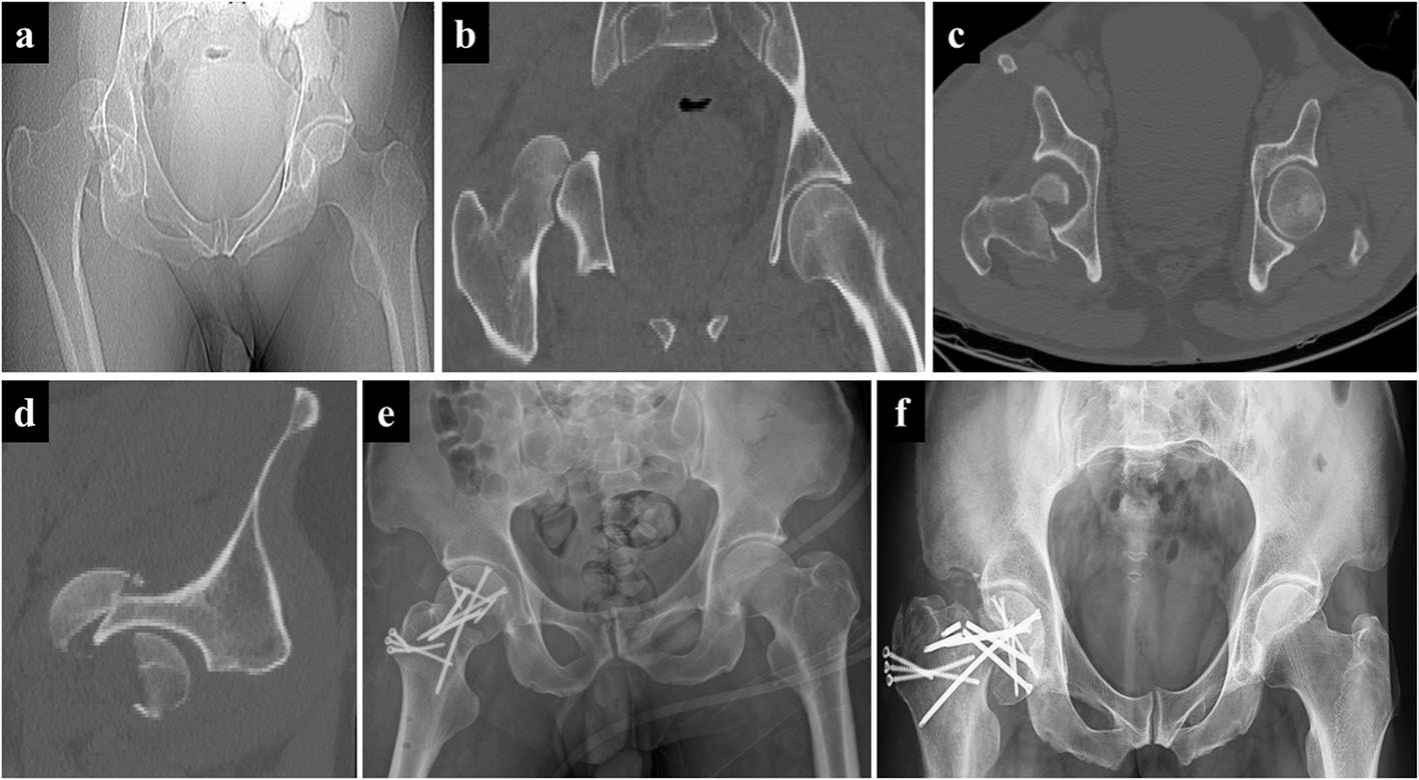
A representative case of the third subtype of the iFHNF, namely posterior dislocation and fracture of the femoral head with postoperative femoral neck fracture. A 43-year-old man involved in a severe traffic accident was diagnosed with femoral head fracture and posterior hip dislocation based on X-ray (a) and CT images (b-d). Open reduction and internal fixation of the femoral head fracture was conducted (e). Finally, the femoral neck refractured without trauma (f)
